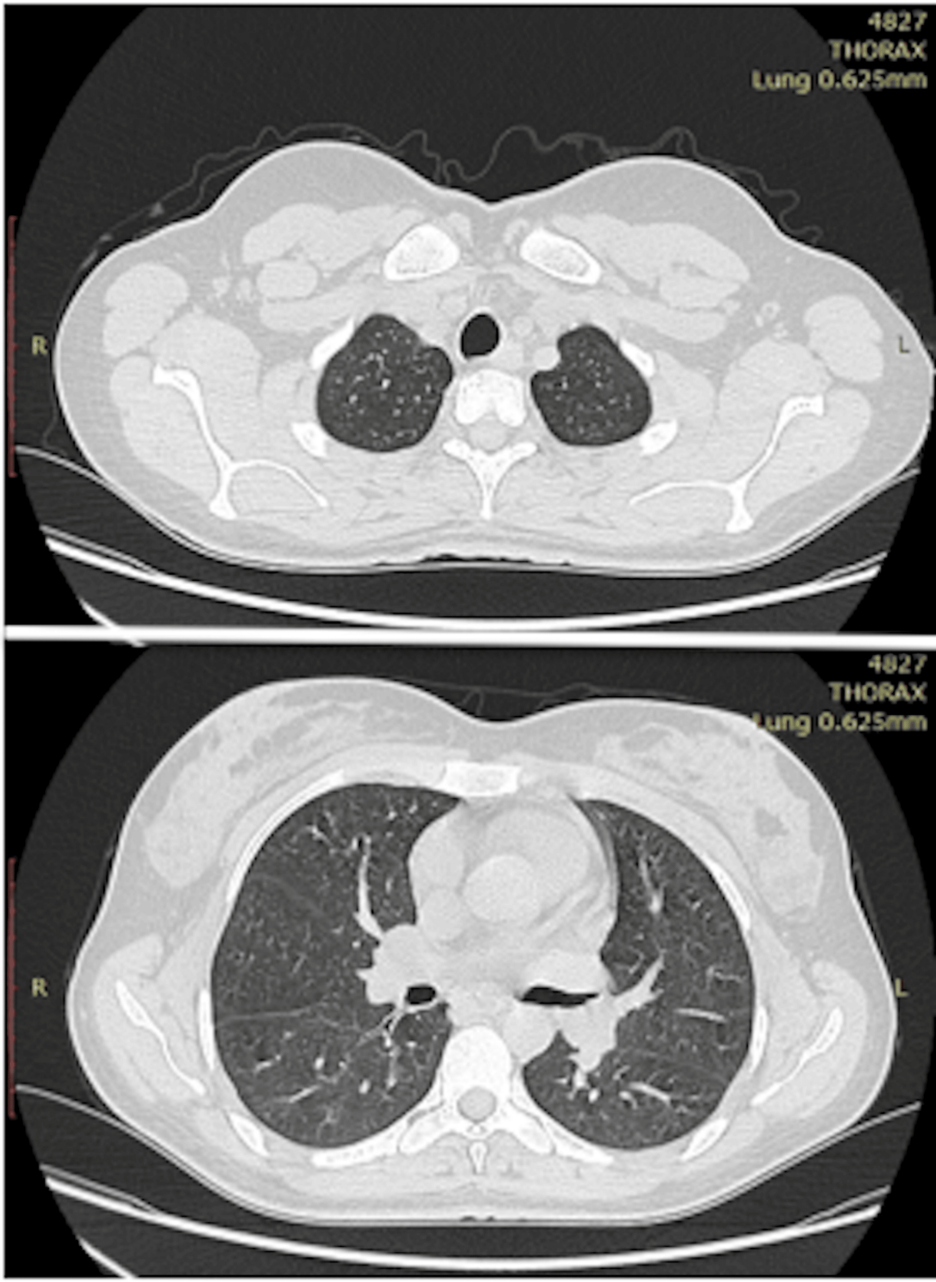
Axial sections of the chest CT show a normal appearance of the lung parenchyma
